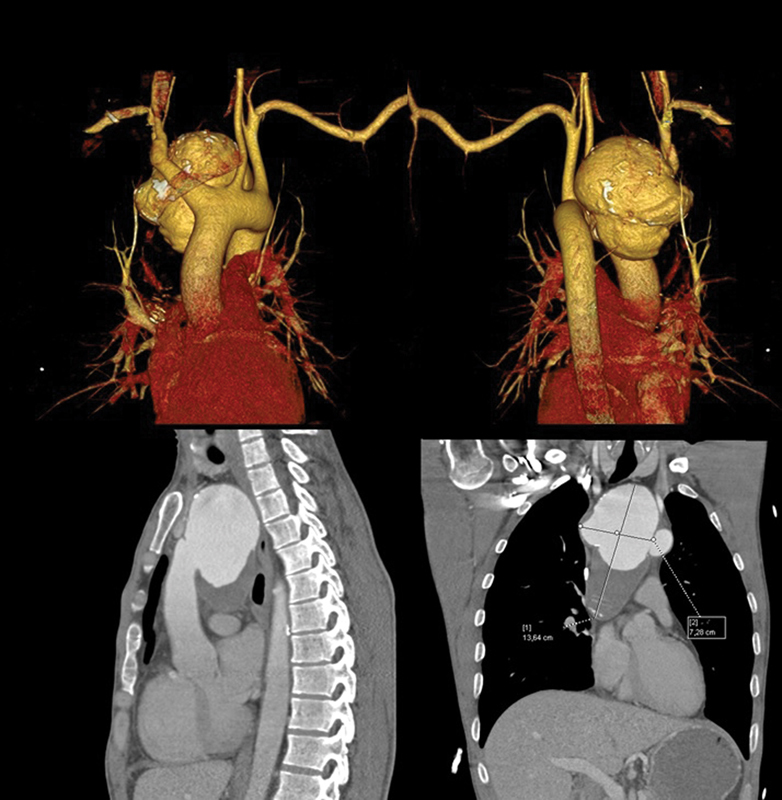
Preoperative CT aortography of pseudoaneurysm of the aortic arch in the posterior mediastinum.
